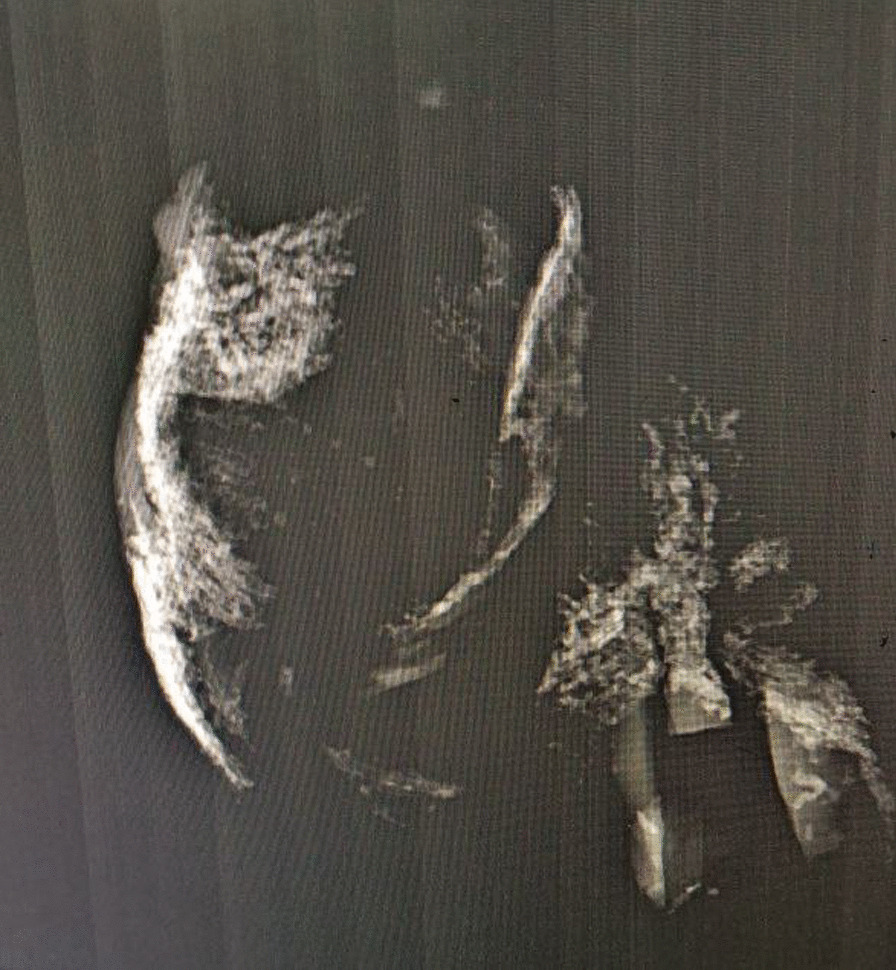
X-ray of the excised hydrocele sac showing extensive calcification of the wall
